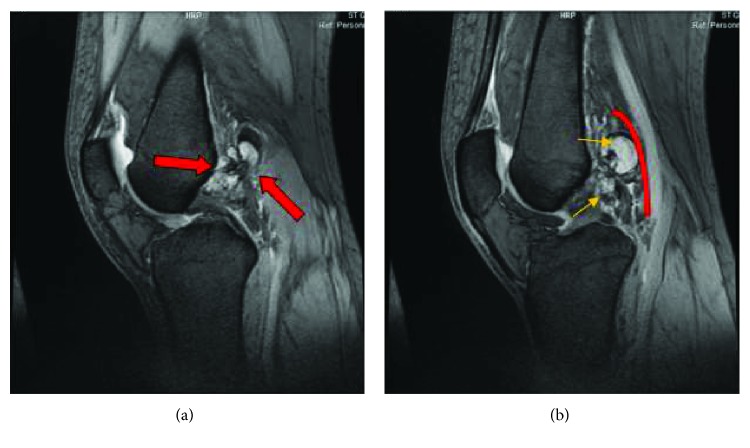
(a) Lateral-view T2 sequence MRI of the left knee showing a multiloculated structure arising from the synovium around the cruciate ligaments within the femoral notch. (b) The lesion is extending beyond the joint capsule posteriorly displacing the popliteal vessels.
